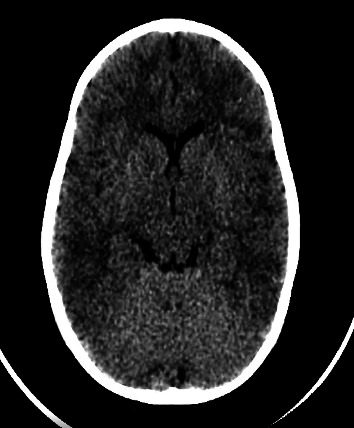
Computed tomography of the brain revealed diffused brain edema with white cerebellum sign.
